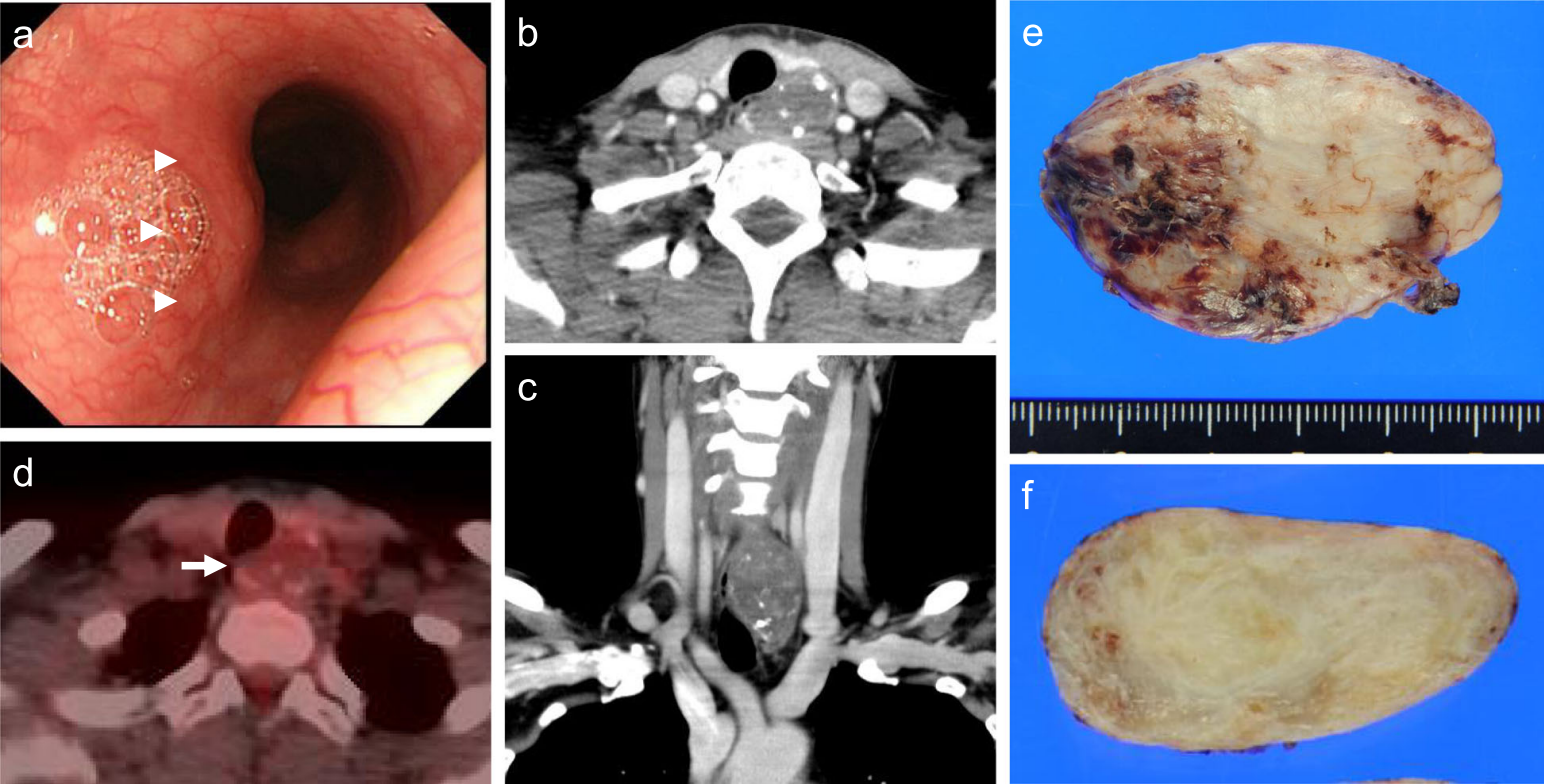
Images obtained before the initial surgery. Esophagoscopy showed a 50-mm-sized submucosal tumor (arrowheads) (a). Contrast-enhanced computed tomography (CT) showed an oval-shaped mass with limited calcification in the cervical esophagus (b, c). ^18^F-fluorodeoxyglucose positron-emission tomography/CT (FDG-PET/CT) showed no FDG uptake in the esophageal tumor (arrow) (d). Macroscopic findings of the initial tumor. It was oval and smooth (e), and its incised surface was yellow–white, homogeneous, and elastic hard (f)
